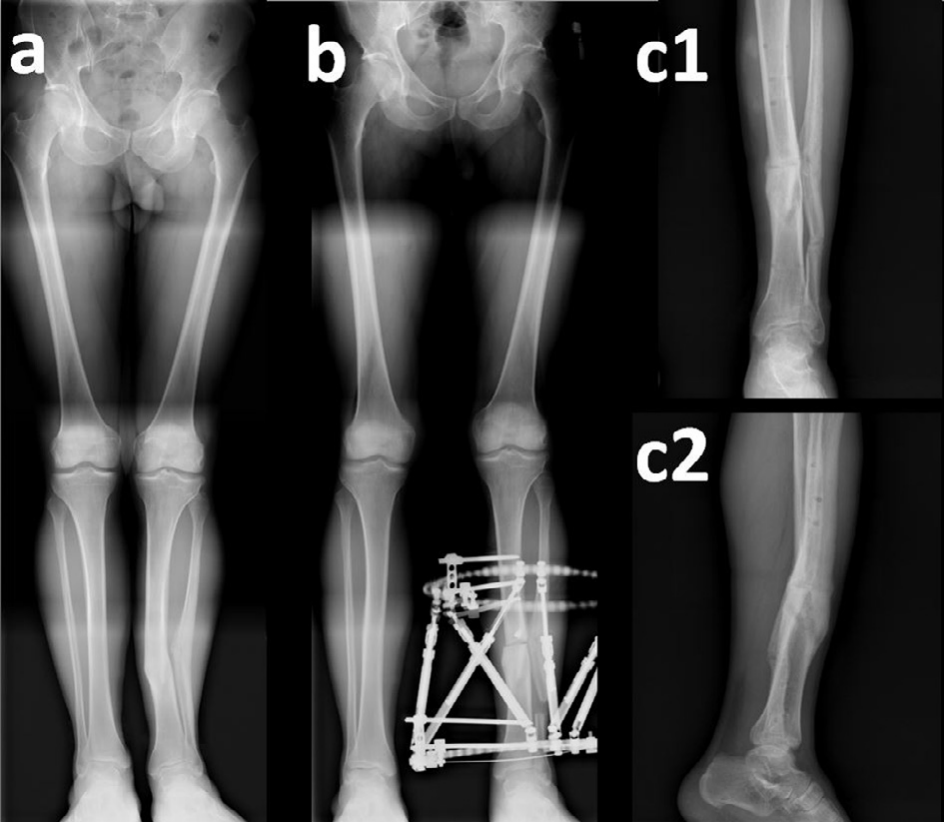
Case No. 17 in Table 1. (a) Valgus, procurvatum and rotation deformity of tibia, (b) after correction with Spider Frame, (c1) and (c2) anteroposterior and lateral X-rays of same patient after removal of Spider Frame.
